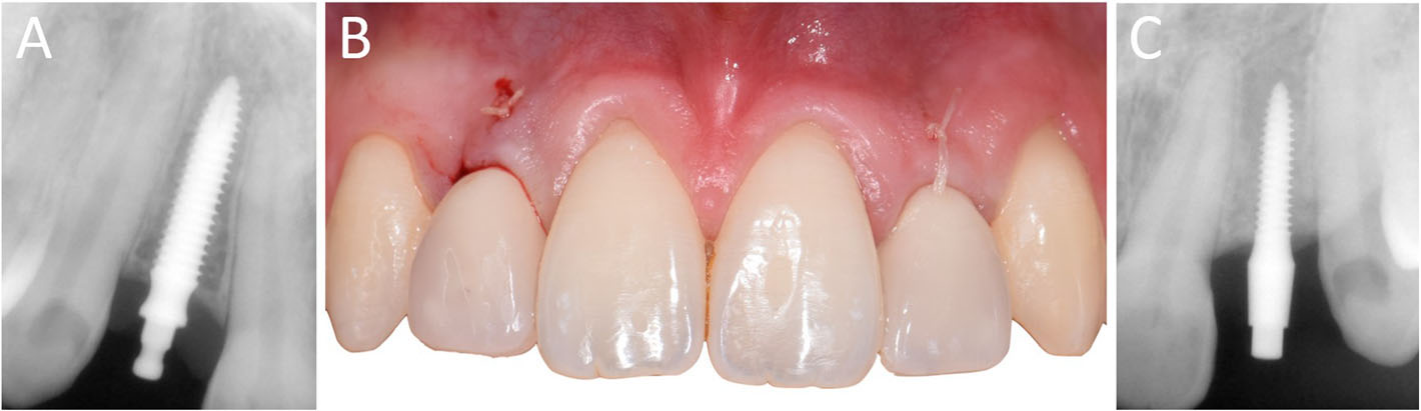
Immediate restoration. The implants are immediately restored by means of single crowns intraorally relined on temporary abutments. a Right lateral incisor, radiographic control immediately after implant placement; b Intraoral picture, frontal view of the provisionals relined on the temporary abutments immediately after implant placement; c Left lateral incisor, radiographic control immediately after implant placement
